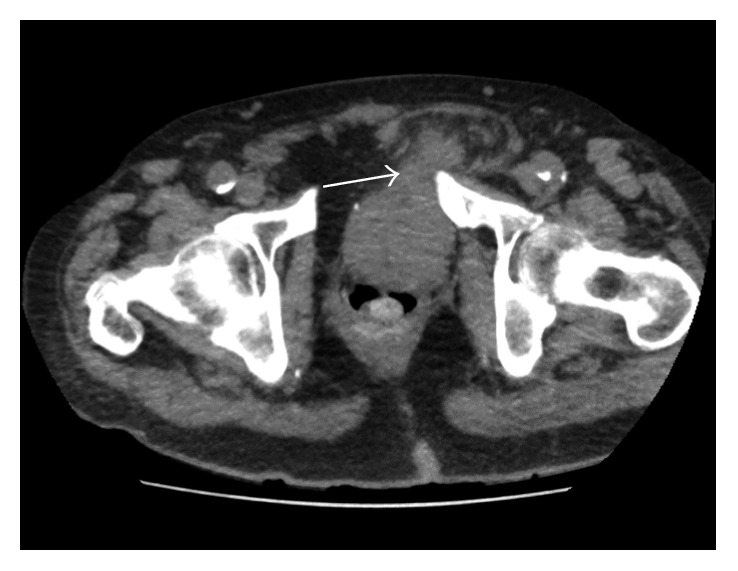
Axial CT scan section showing herniation of the bladder into the left inguinal region.
